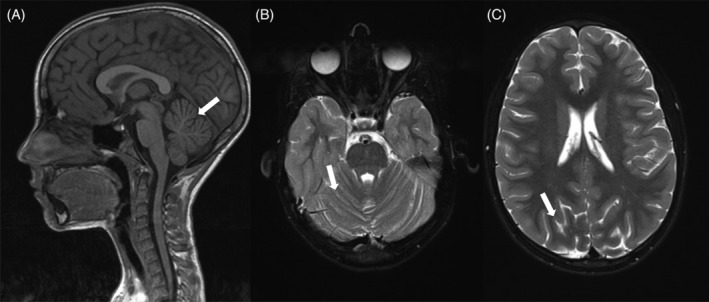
A‐B, MRI images, taken at age 8. Cerebellar atrophy is visible on T1 and T2 weighted images (arrow). C, In addition, subtle atrophy of the supratentorial cortex can been seen (arrow). MRI, magnetic resonance imaging
